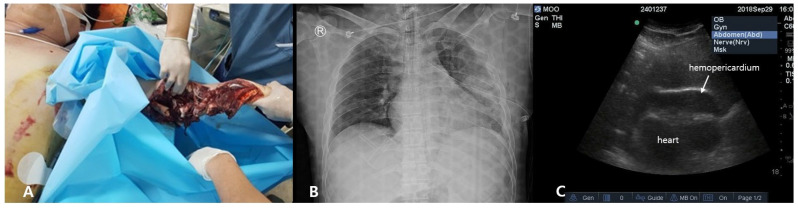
Initial presentation. (A) Mangled left arm. (B) Initial chest radiograph. (C) FAST showing hemopericardium.
